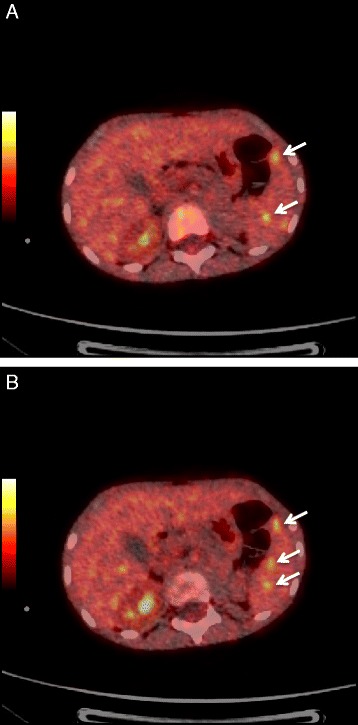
Panels (a) and (b) showed fused images of [^18^F]FDG-PET/CT (see white arrows) corresponding to focal hypermetabolic areas in spleen (highest was SUV_max_ = 3.2), previously visualized as hypointense images on computed tomography. Scanning was performed with 90 kV, 10–120 mAs (smart mA) and 3.75 mm section thickness. Acquisition time was 2 min per table position with 10-slice overlap at the FOV borders. PET image data sets were reconstructed iteratively (4 iterations and 24 subsets) by applying the CT data for attenuation correction. Fused images were displayed on a workstation using dedicated software
